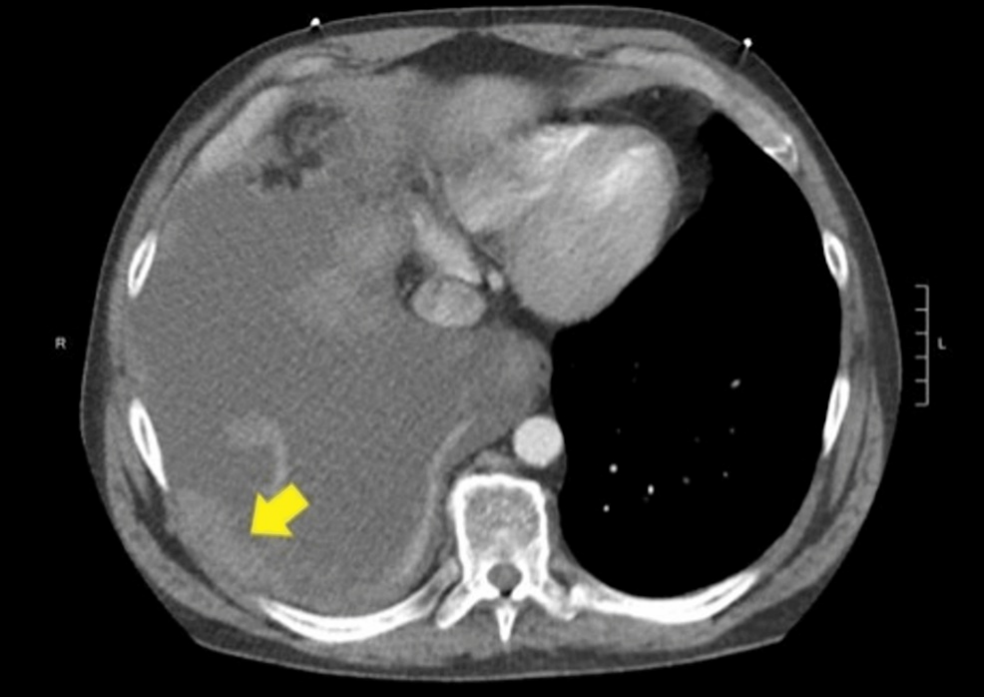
Chest CT with intravenous contrast on presentation. Large right-sided pleural effusion and nodular pleural mass (yellow arrow).
